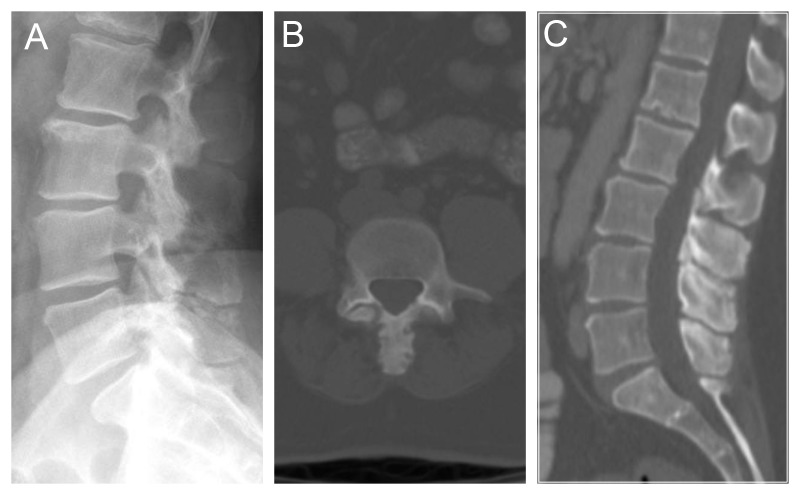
Radiographic findings on plain film and CT Mild lumbar spondylosis with prominent, hypertrophic spinous processes, contacting one another between L3 and L5 with adjacent sclerosis.
